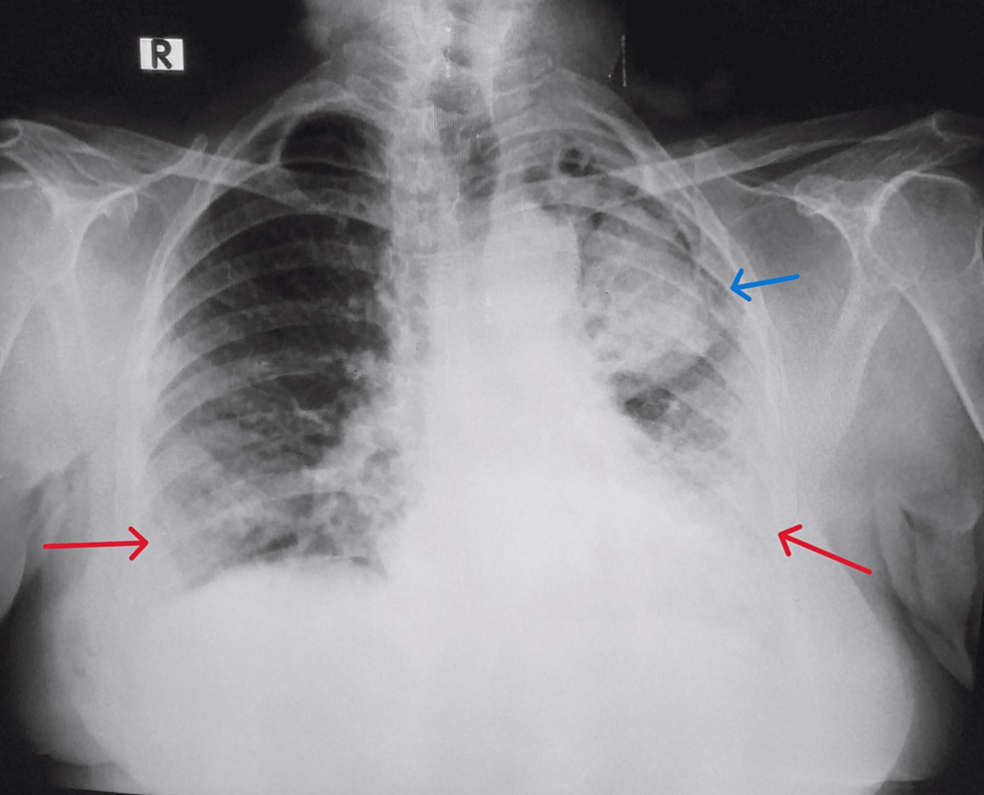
Chest radiograph revealed a tracheal shift to the left, a cavity in the left upper and mid-zone with intracavitary space-occupying lesion (blue arrow), bilateral mid and lower zone non-homogenous reticulonodular opacities with haziness (red arrows), and crowding of ribs were noted.
